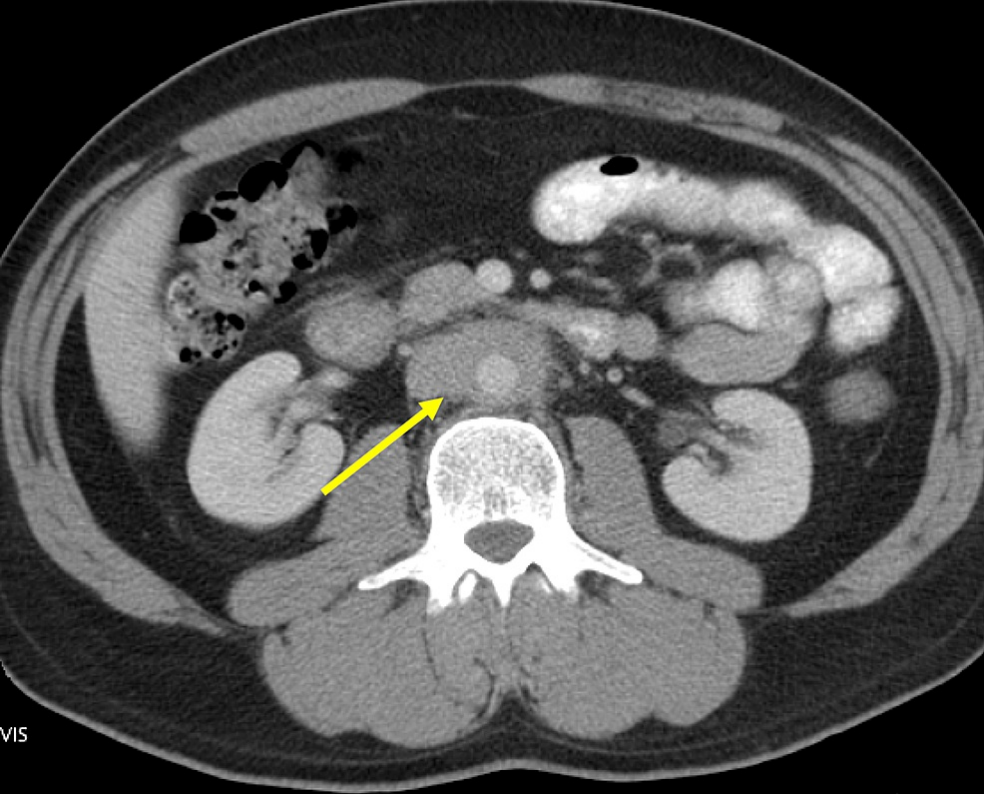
CT that was used to diagnose lymphoma. Visible is a periaortic infiltrating mass (arrow) and mildly reactive lymph nodes. Note that lymph nodes are non-confluent, the aorta is not displaced anteriorly, the bones are intact, and there is no psoas muscle dislocation.
